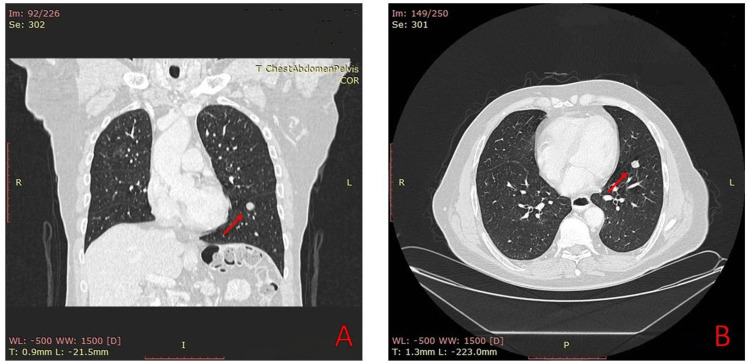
Chest CT revealed a 1 cm lesion in the lower lobe of the left lung (red arrows).
